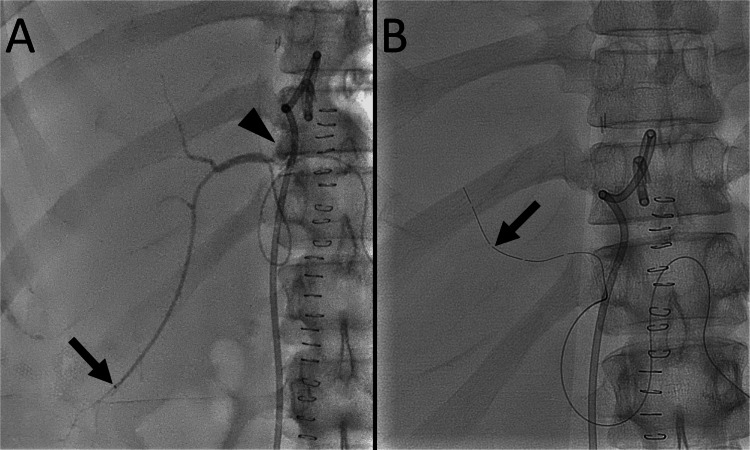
Percutaneous biliary drainage tube placement in the right posterior segmental bile duct (A) A frontal radiograph with contrast injected using a microcatheter (arrow) advanced to the segment 6 bile duct shows leakage of the contrast medium (arrowhead) into the biloma. (B) A frontal radiograph shows a drainage tube (arrow) placed in the segment 7 bile duct.
